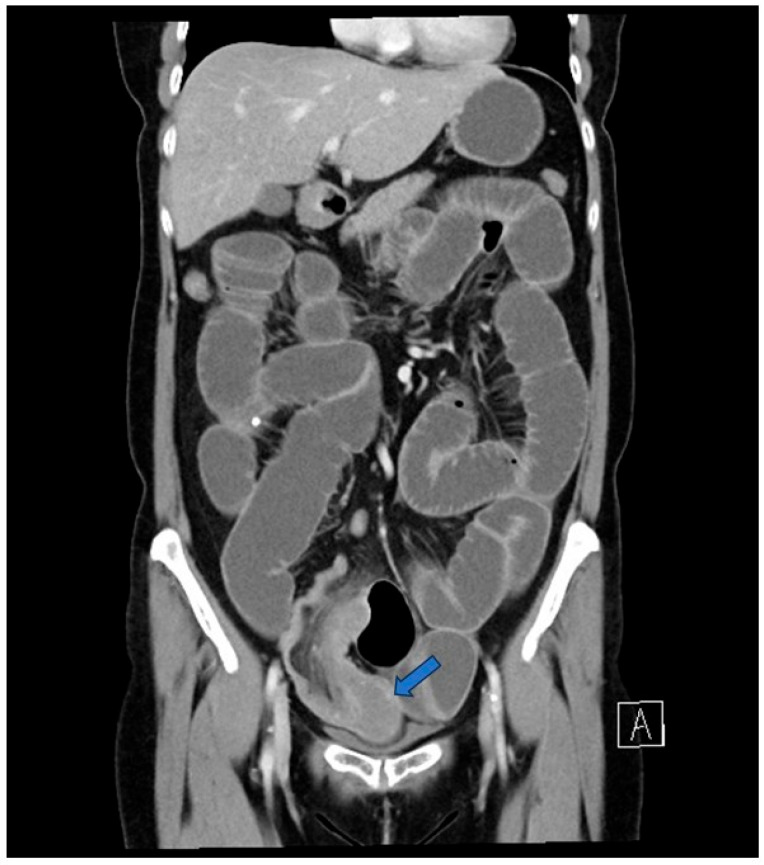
Coronal contrast-enhanced CT image demonstrating ileo-ileal intussusception with a rounded intraluminal lead point (arrow) and associated upstream small-bowel obstruction.
